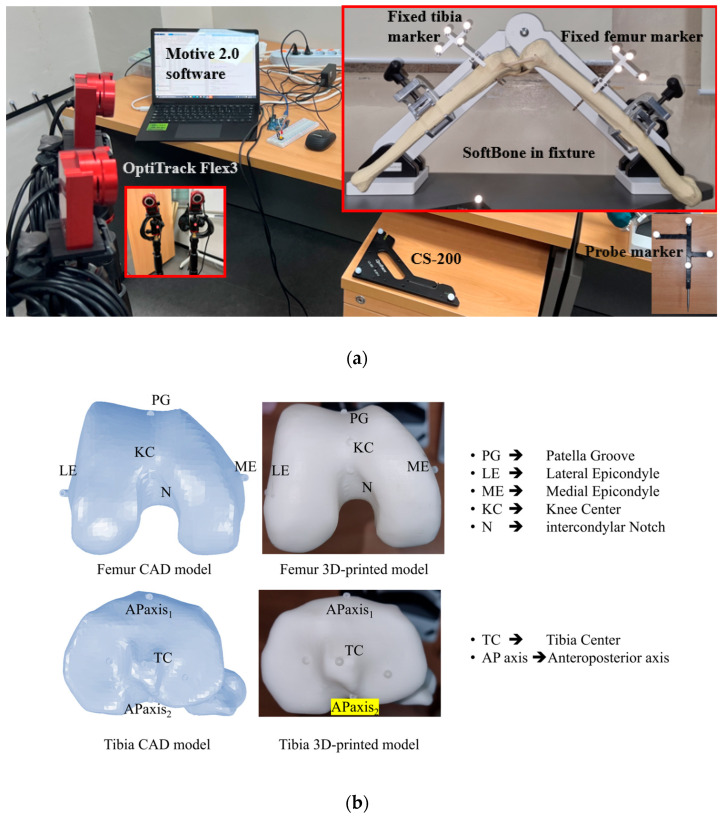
(a) 3D printed bone models, (b) CT scanned CAD bone.
